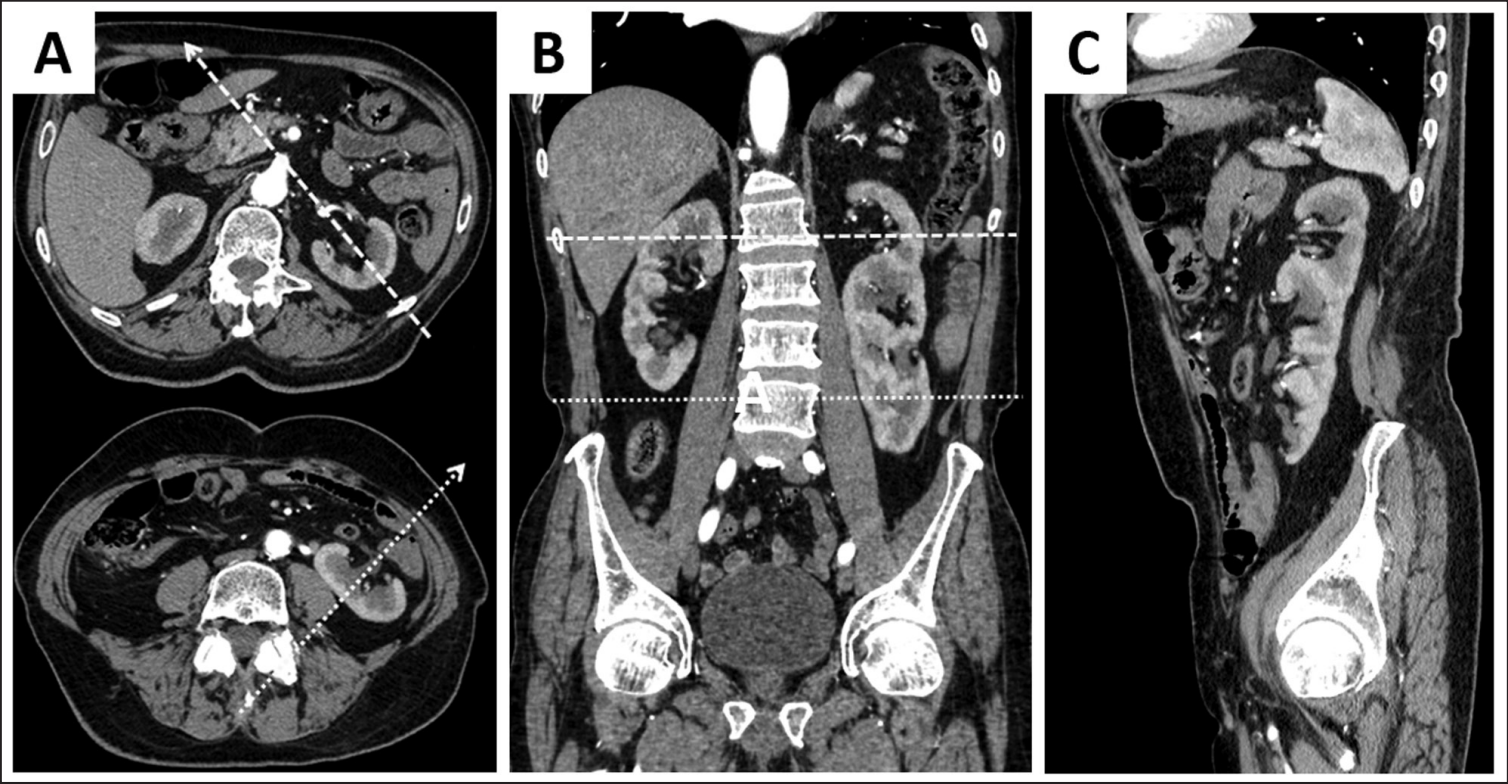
Contrast-enhanced CT with axial (A), coronal (B) en sagittal (C) reformations demonstrating a large, S-shaped kidney in the left renal fossa and a normal kidney on the right.
